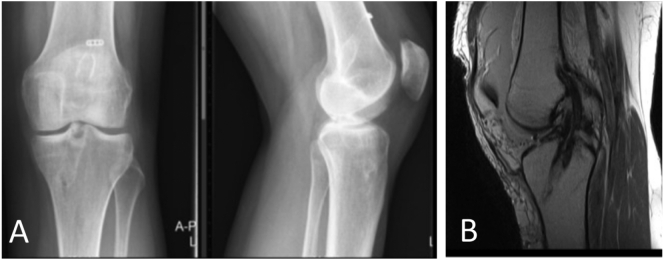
A. Plain X-rays AP and lateral left knee (patient 2). Posterior position of the tibial tunnel, steep orientation of the graft. B. Sagittal MRI image of an intact ACL graft (patient 2).
